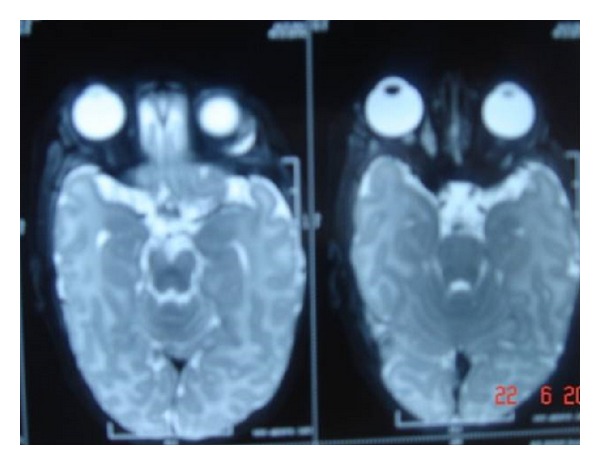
MRI scan showing lesion in the orbit.
